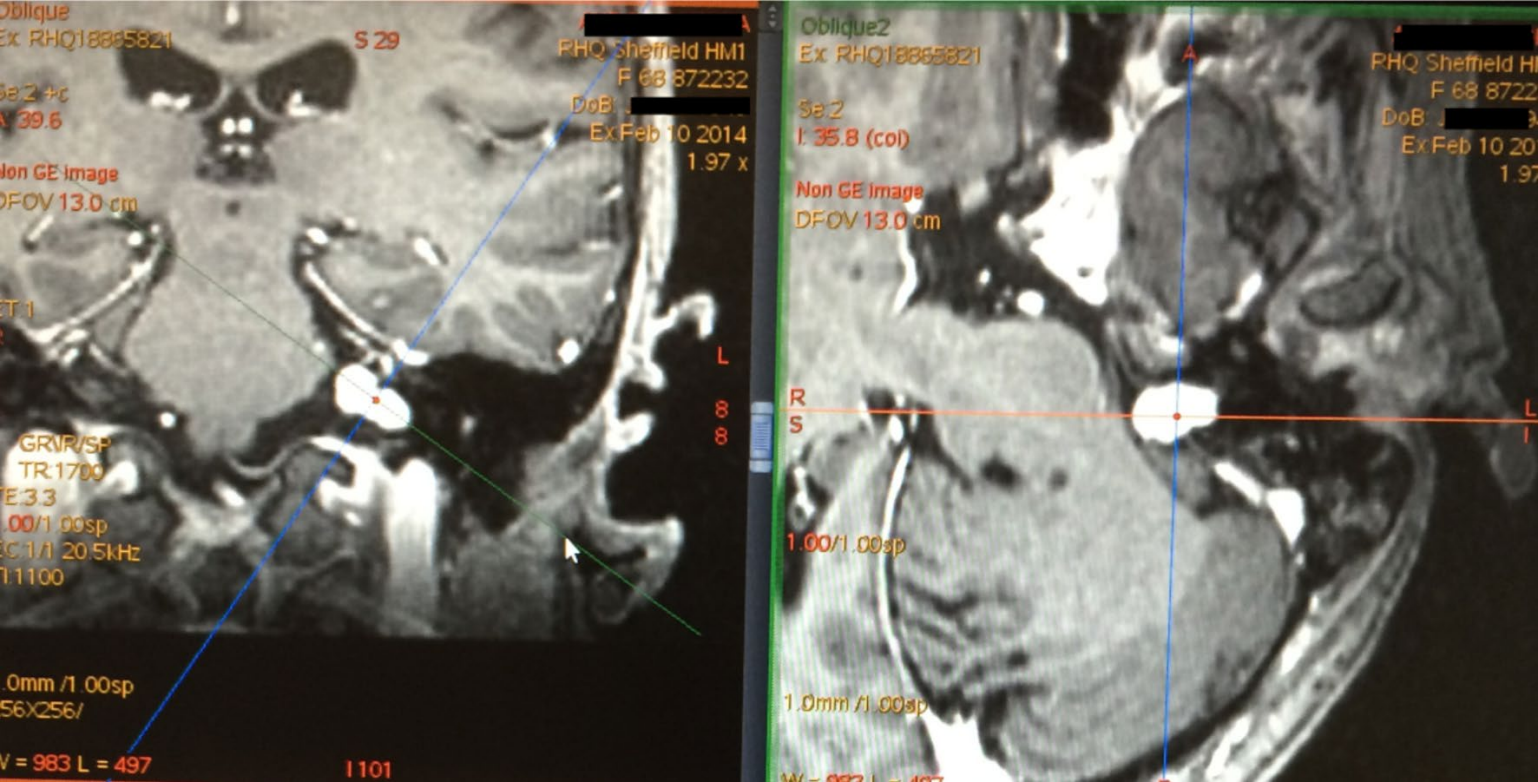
MRI of left VS showing long axis of tumour at 40° angle to the horizontal when viewed in coronal plane (left image), with the obliquely reformatted axial image displayed on the right
